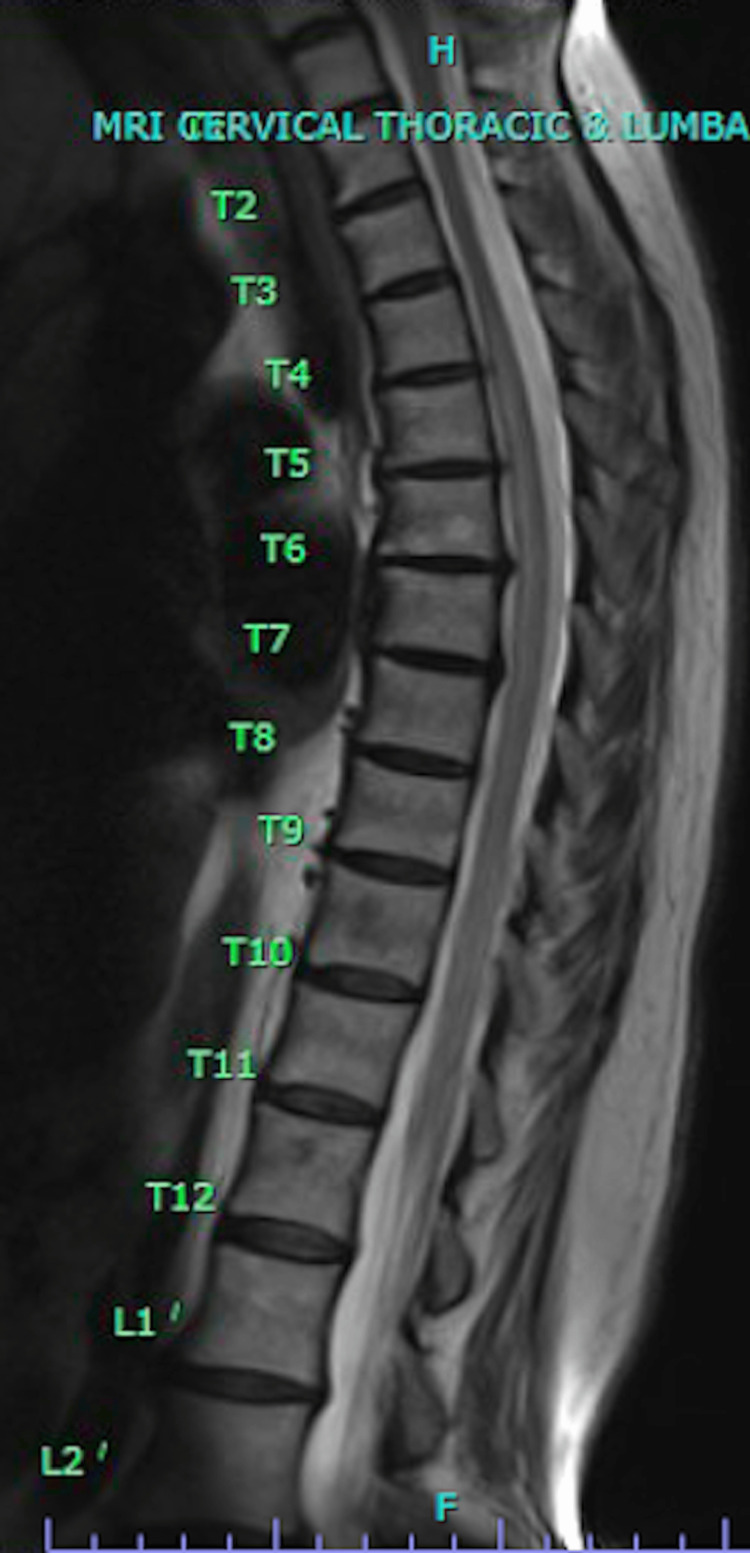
MRI of the T-spine without contrast reveals enhancement of the spinal cord from T9-T10 and T11-T12, suggestive of spinal cord infarct or transverse myelitis
